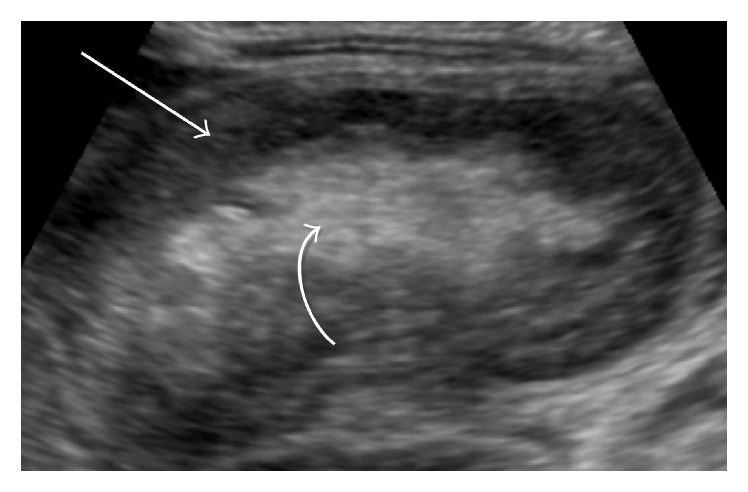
Longitudinal oblique abdominal ultrasound in the midline demonstrates an ovoid mass (curved arrow) showing the characteristic “pseudokidney” feature of intussusception.
